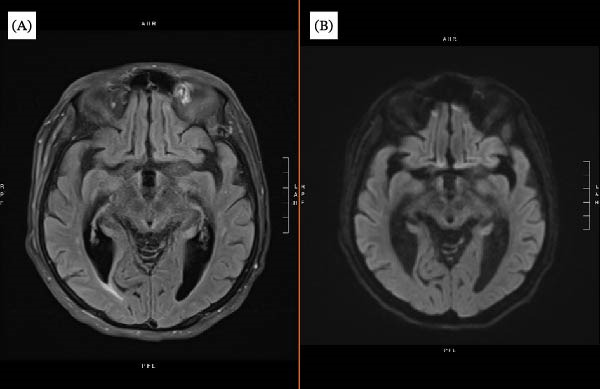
Follow‐up brain MRI demonstrating subtle interval temporal lobe signal abnormality in the setting of CSF‐confirmed HSV‐1 encephalitis. (A) Axial T2‐FLAIR image and (B) axial diffusion‐weighted image (DWI) at the temporal lobe level show subtle interval signal abnormality compared with the admission MRI.
